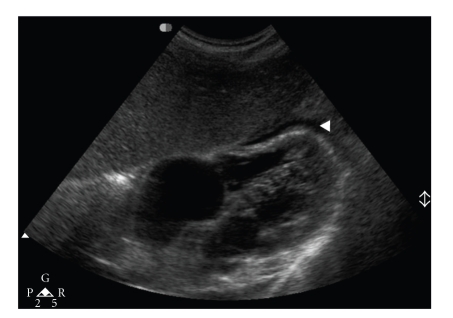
Ultrasonography of abdomen showed a minimal pericardial effusion.
